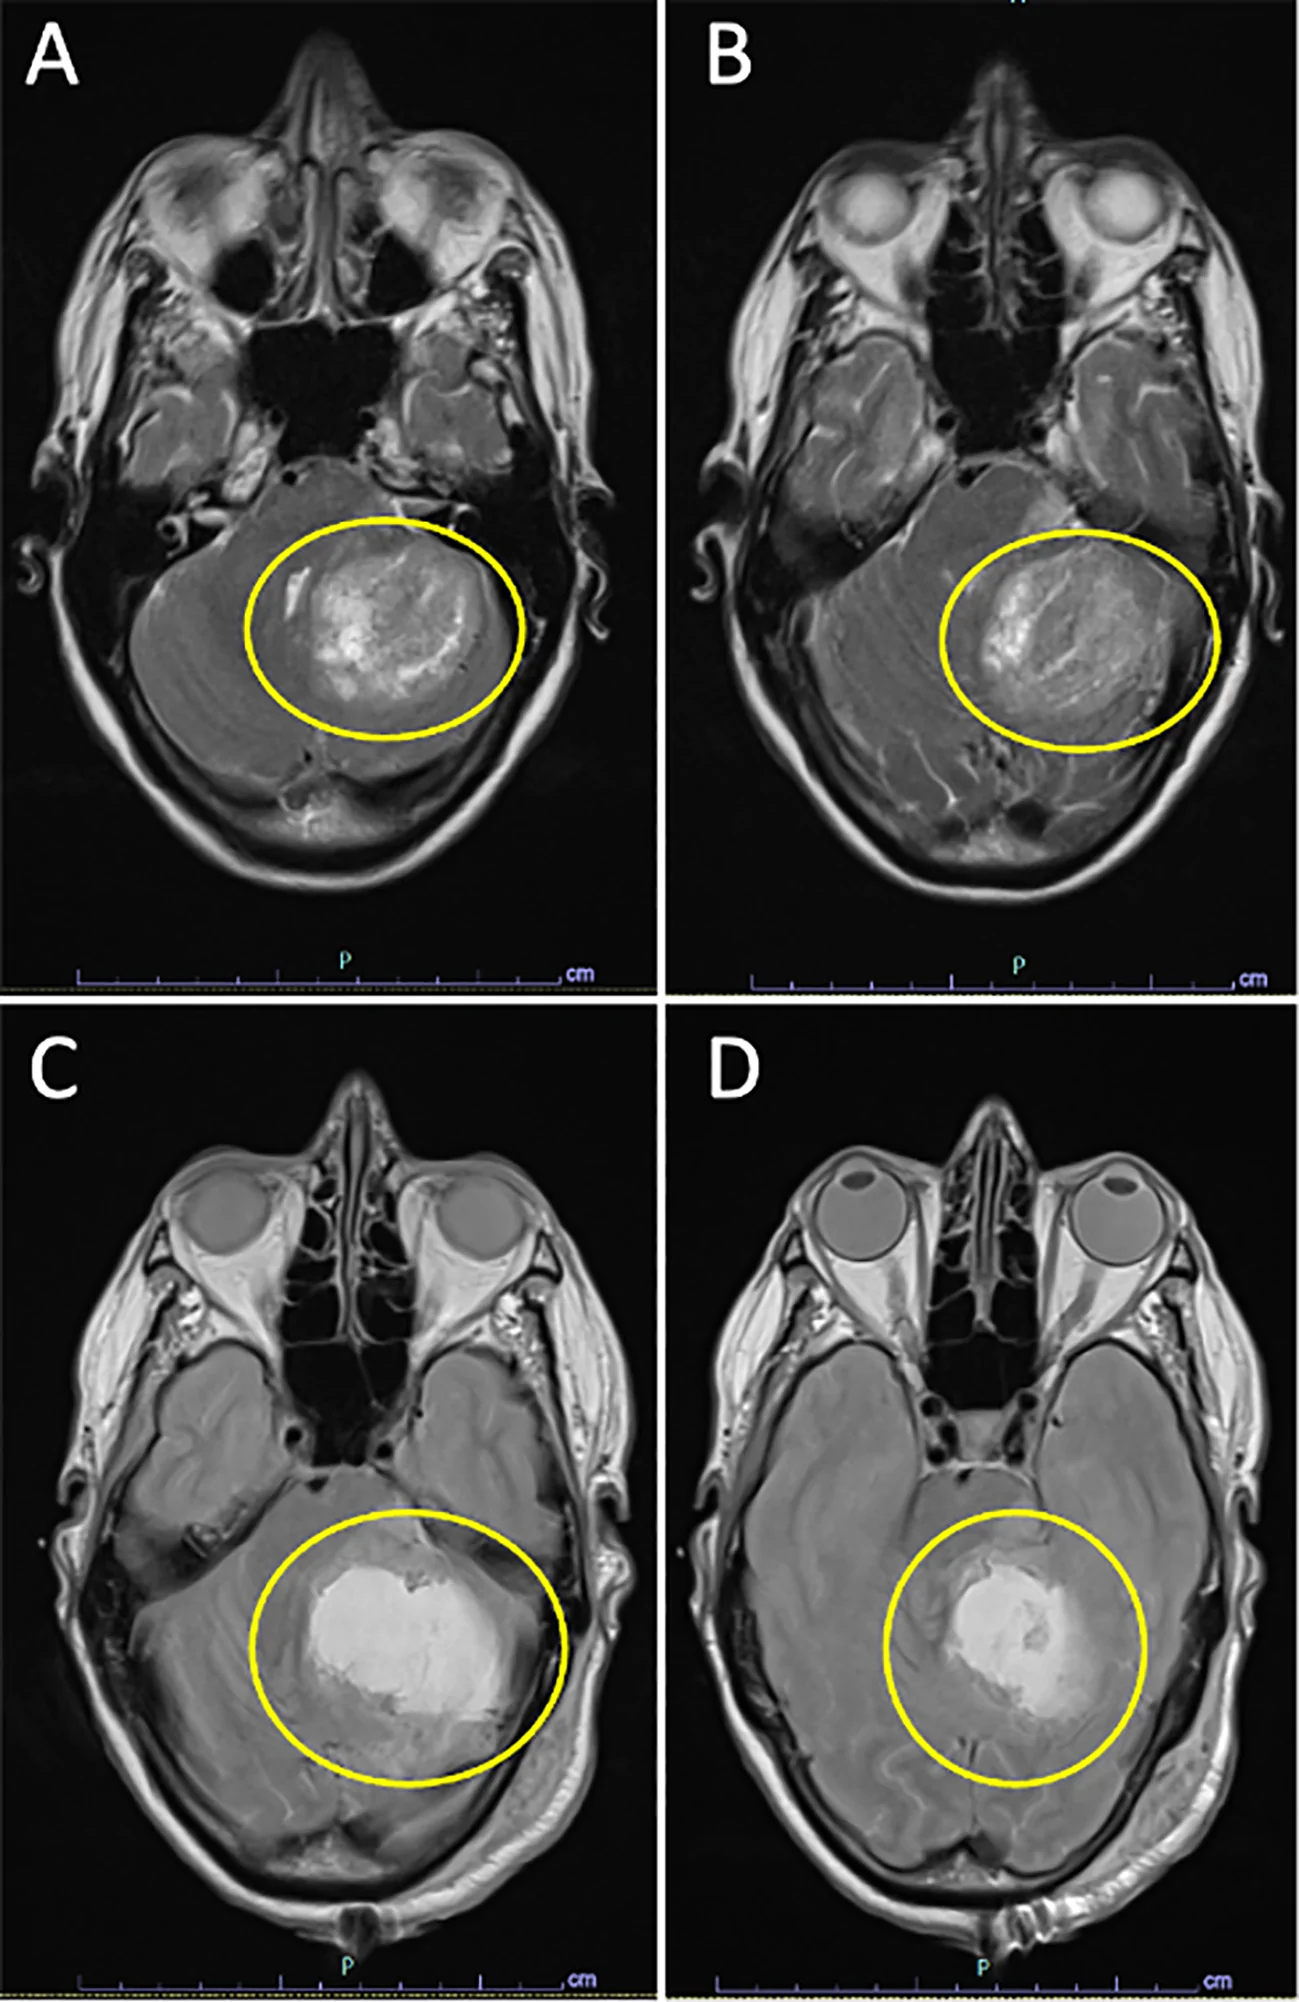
Cranial MRI (A–D) showing a left cerebellar intra-axial lesion (4 x 2.5 cm) with extension into the left cerebellar peduncle and brainstem. Yellow circles indicate the cerebellar lesion.
